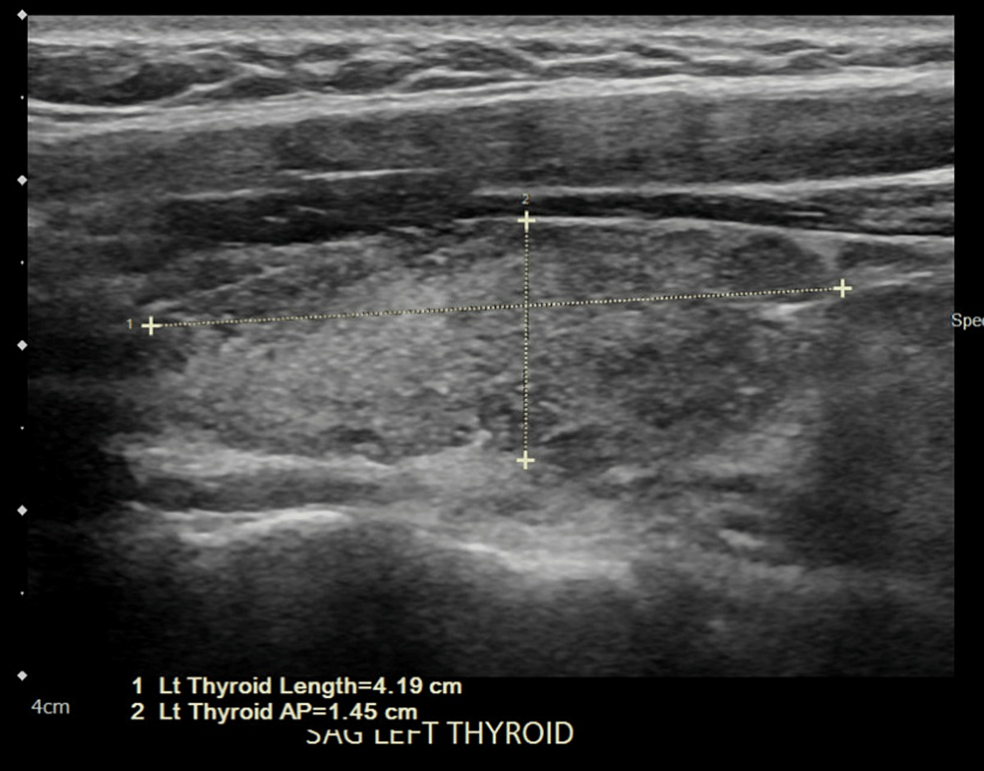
Ultrasound of the right thyroid lobe showing an enlarged heterogeneous thyroid gland
